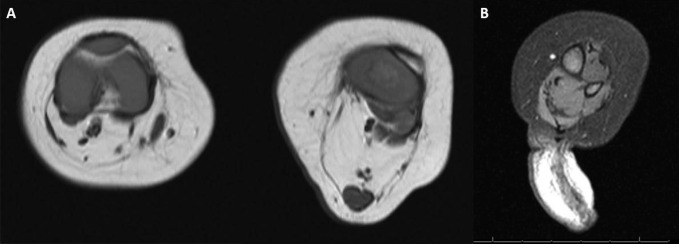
A, MRI of the left leg demonstrating the source of the pterygium to be a long biceps femoris tendon. B, MRI of left leg showing the toe-like skin tag on the posterior aspect of the calf.
